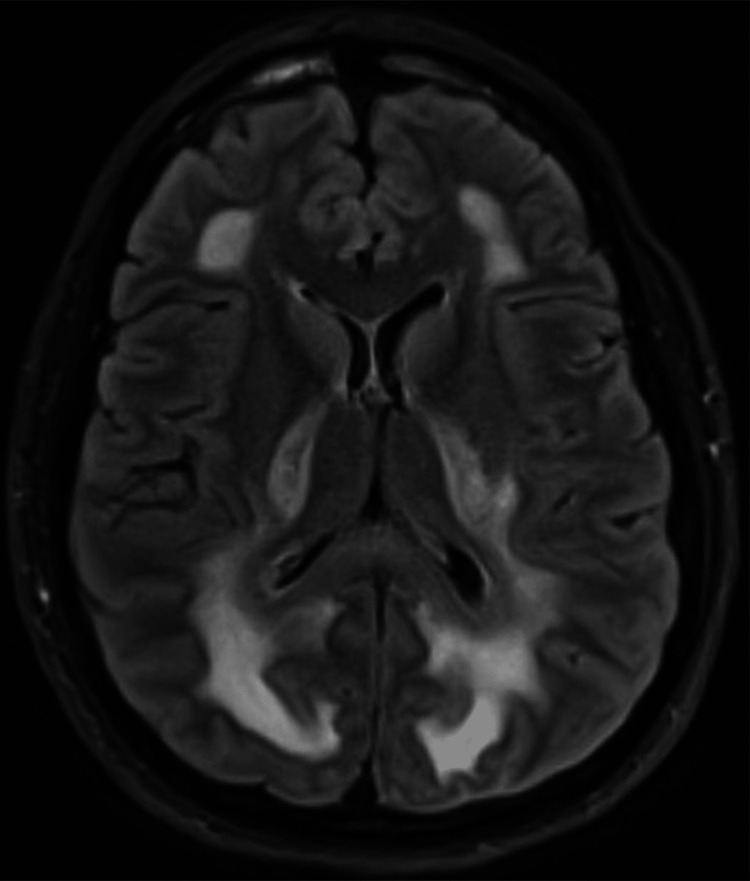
FLAIR sequence from the patient’s initial MRI demonstrates abnormal hyperintense signal in the white matter of the frontal lobes, in the posterior limb of the internal capsule, and extending into the white matter of the parietal and occipital lobes. There is sparing of the subcortical U-fibers and the deep gray nuclei. FLAIR: fluid-attenuated inversion recovery
